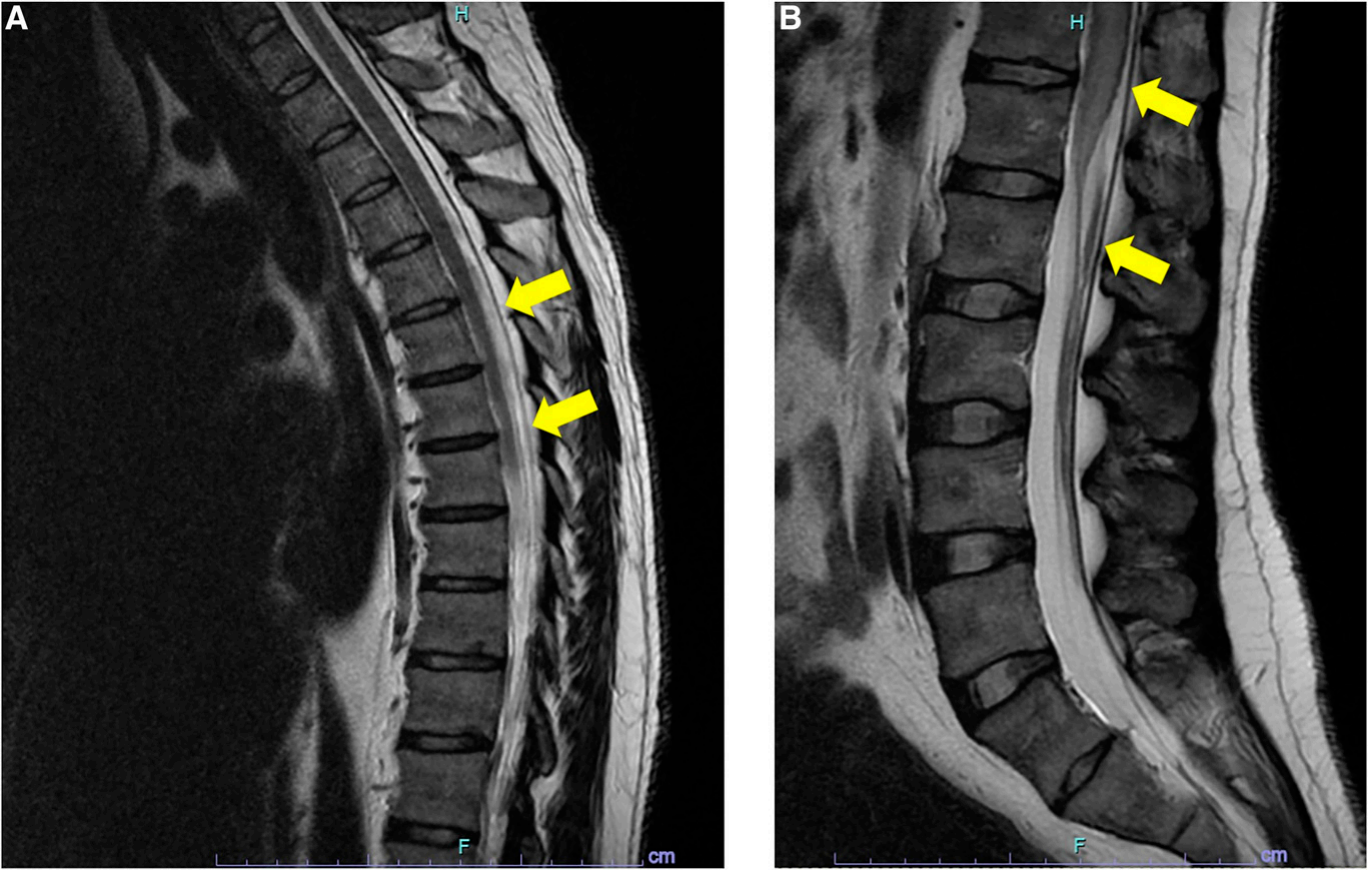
Initial MRI with contrast showing features of transverse myelitis. (A) Hyperintense T2 signal from T8 to the conus medullaris (arrows) and (B) edema of the conus medullaris with heterogenous enhancement post-contrast (arrows). The cause was probable neuroschistosomiasis, an inflammatory response to schistosomal ova deposited in the spinal cord. This figure appears in color at www.ajtmh.org.
